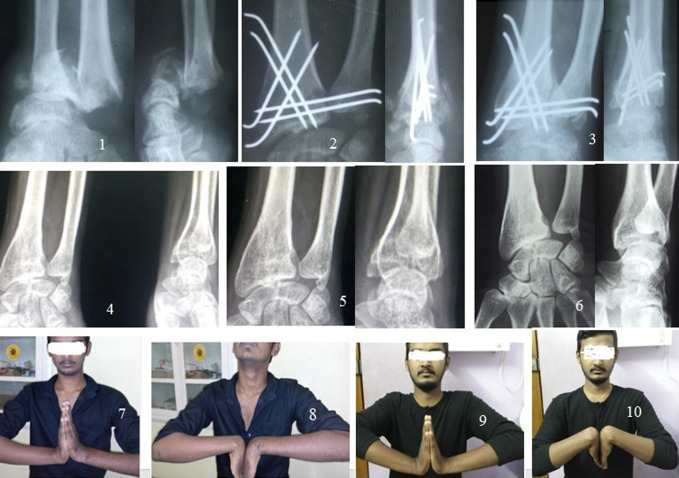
Photograph showing a 21-year-old man from day 1 to 3 years (left wrist). A, Pre-op radiograph. B, Post-op radiograph. C, radiograph at 6 weeks. D, radiograph at 3 months. E, radiograph at 6 months. F, radiograph at 3 years. G, Dorsiflexion at 3 months. H, Palmar flexion at 3 months. I, Dorsiflexion at 3 years. J, Palmar flexion at 3 years.
